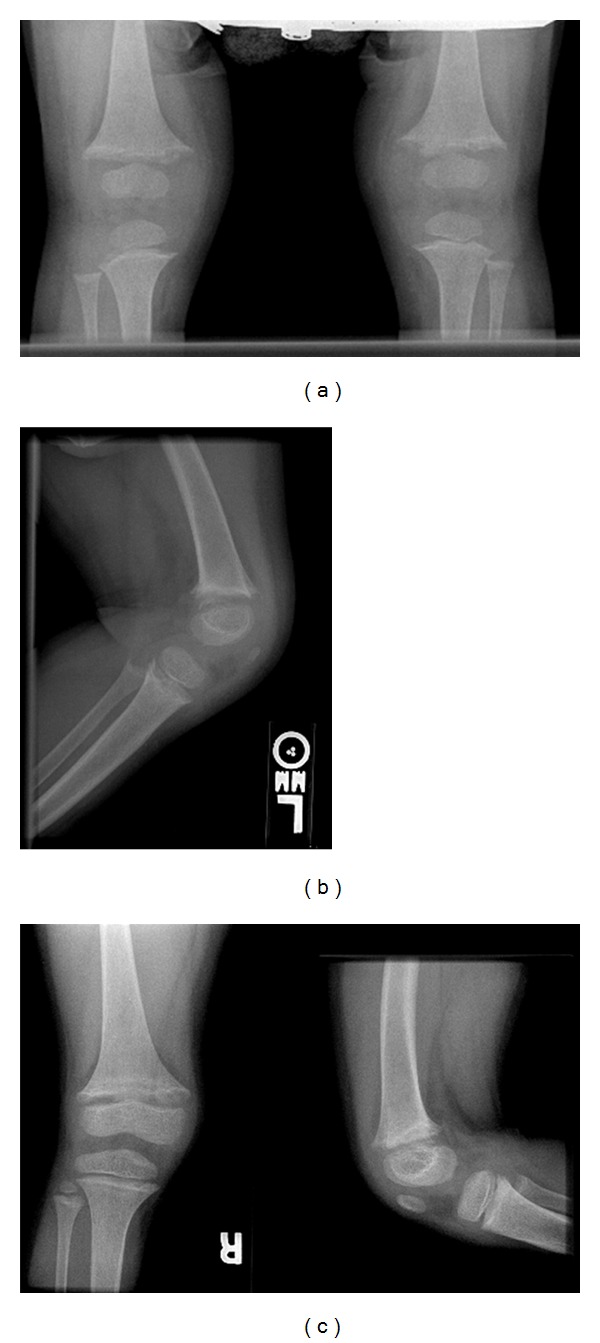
Radiographs of knees at diagnosis (a) and before phosphate and calcitriol were commenced. (a) Radiograph of both knees at 1 year of age showed irregularity, fraying and flaring of the distal femoral and proximal tibia metaphyses bilaterally. (b) Radiograph of knees at 3 years of age showed worsening rickets with increased widening, cupping and fraying of metaphyses of the distal femur, the proximal tibia, and fibula. (c) Radiograph of knees at 4 years of age showed persistent widening of metaphyses in both knees.
